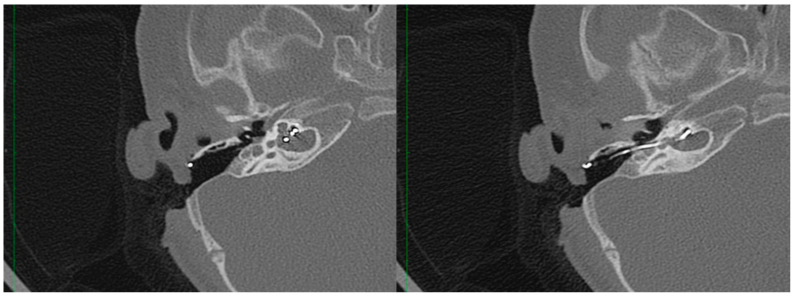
Intraoperative CT scan image from patient 5. The electrode array is located partially in the basal turn but also in the fundus of the IAC.
